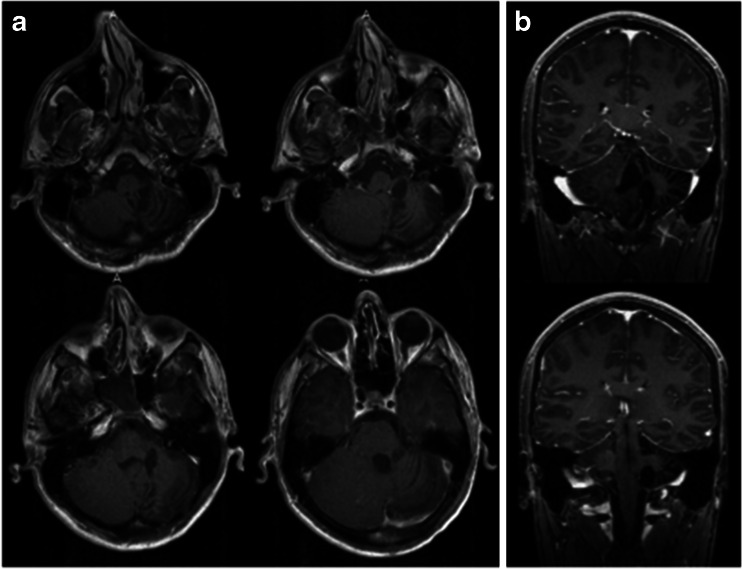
Case 4. Post-operative T1-weighted postcontrast MRI (a axial, b coronal). There is no evidence of disease
